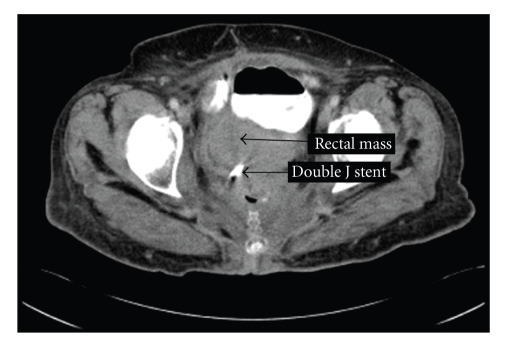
CT scan of pelvis showing rectal mass and double J stent.
